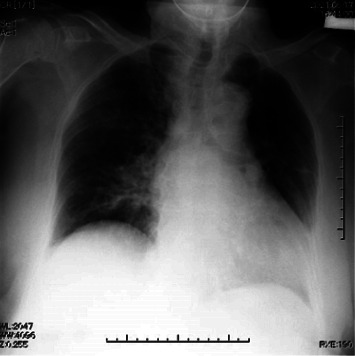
Chest radiograph at 4 hours after surgery.
